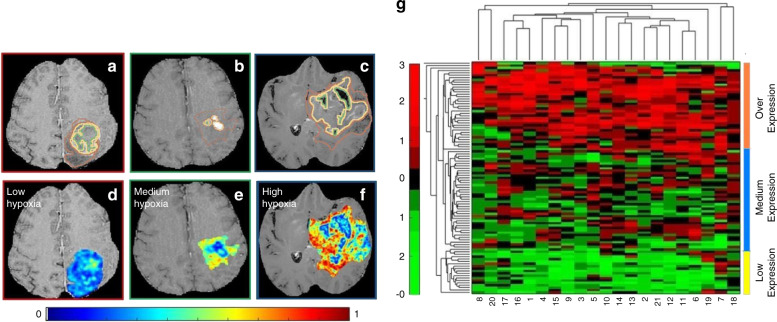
Construction of Hypoxia Enrichment Score. a–c show a 2D Gd-T1w MRI slice with expert-annotated necrosis (outlined in green), enhancing tumour (yellow) and oedematous regions (brown) in three different GBM patients that exhibited low, medium, and high hypoxia enrichment score (HES) respectively. The corresponding inverse difference moment (Haralick) feature map has been overlaid on the manually annotated tumour regions, for HES_low_ (d), HES_medium_ (e), and HES_high_ (f). g Unsupervised clustering of the RNAseq data from the 21 hypoxia associated genes clustered as high hypoxia (HES_high_—shown in navy blue, medium hypoxia (HES_medium_—shown in teal) and low hypoxia (HES_low_—shown in yellow). The *x* axis in the clustergram represents the 21 genes and *y* axis represents the patient population of 97 GBM cases. Figure from Beig et al.^87^; licensed under a Creative Commons Attribution (CC BY) license.
